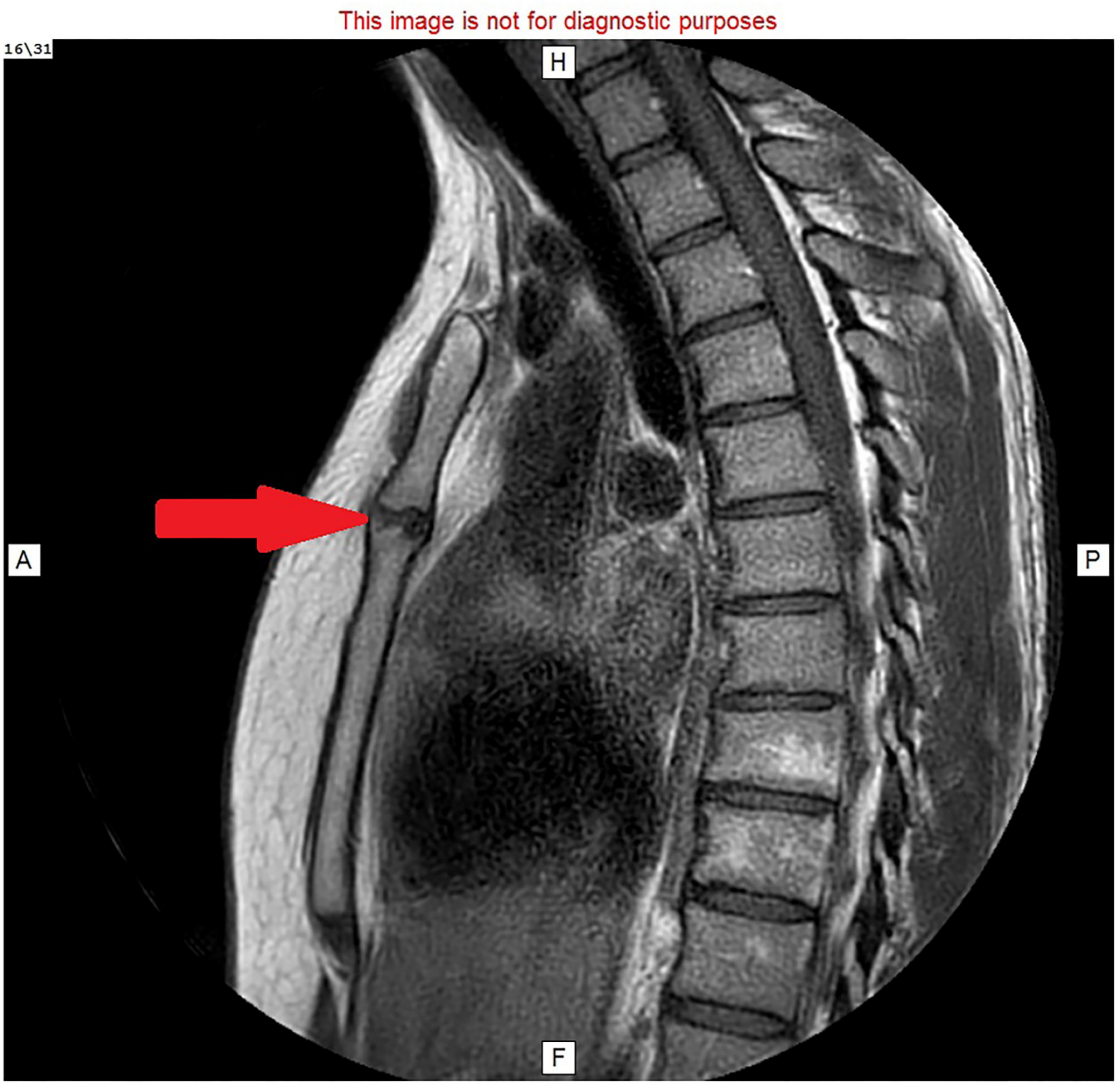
MRI image: T1 with faset and gadolinium contrast, sagittal view, showing hyperemic fluid in the manubriosternal joint (red arrow).
